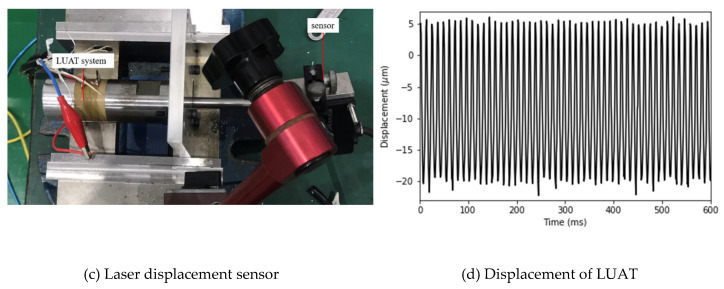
Displacement testing experiment. (a) longitudinal ultrasonic assisted turning (LUAT) system; (b) layout of displacement testing experiment; (c) laser displacement sensor; (d) displacement of LUAT.
